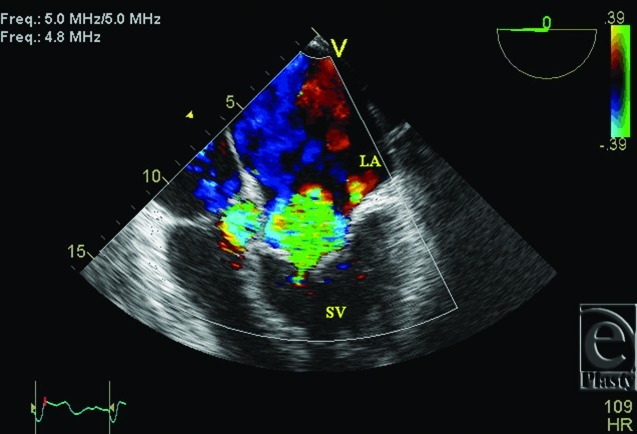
Transesophageal echocardiogram color Doppler showing severe systemic atrioventricular valve regurgitation.
